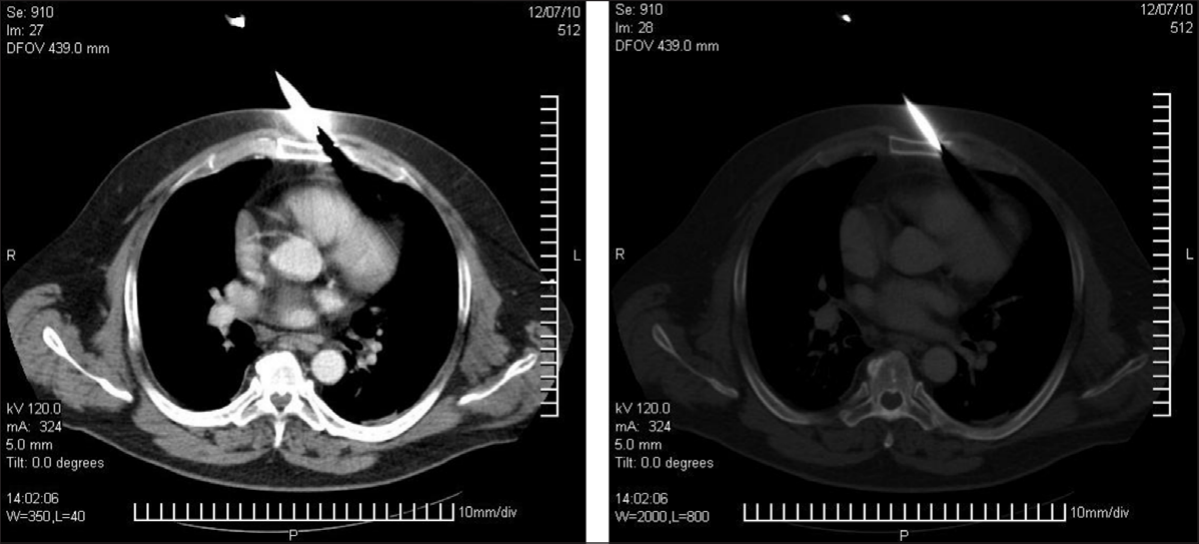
Chest CT study with contrast. A foreign metallic body (knife) causes a sternum fracture, well visible in the axial planes re-constructed with a parenchymal window (a) and, especially, with a bone window (b).
